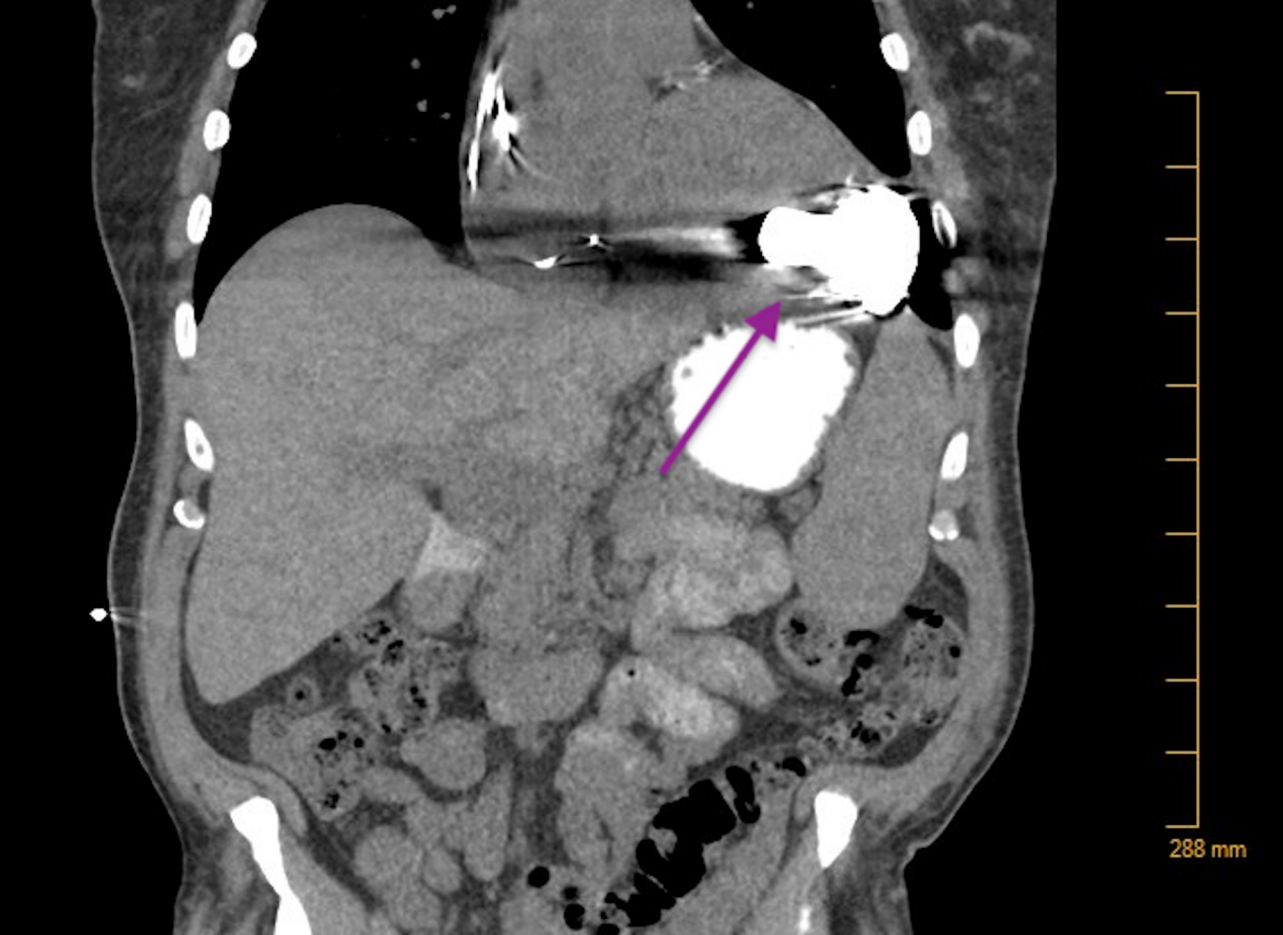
CT of the Thorax No fluid collection noted around the pump.
